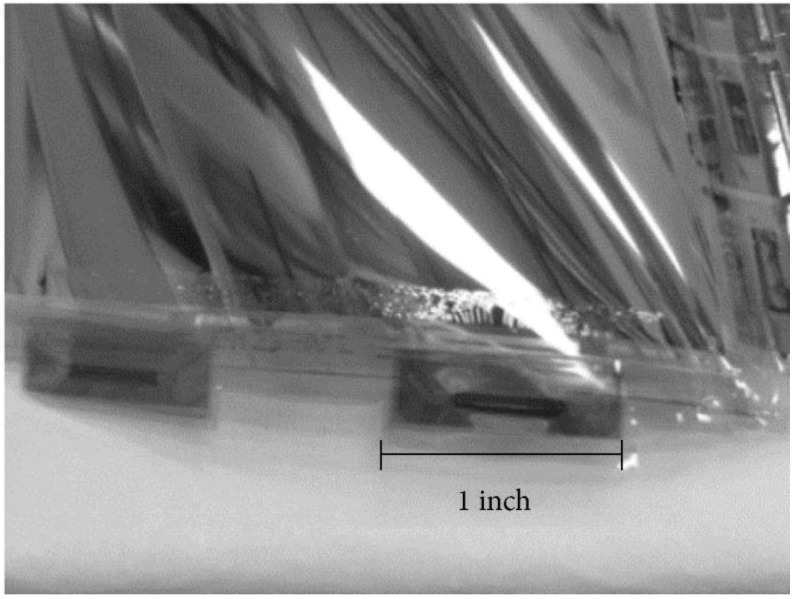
Full fill factor digital X-ray detector backplane showing stress related failure due to high intrinsic film stress in the i-Si layer.
